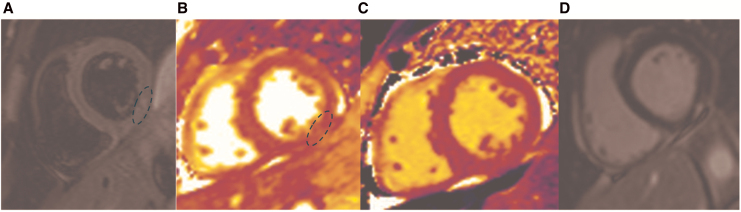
Follow-up cardiac magnetic resonance imaging. Follow-up CMR showed preserved ventricular size and systolic function, substantial reduction of myocardial oedema compared with baseline, and persistent focal non-ischaemic subepicardial late gadolinium enhancement consistent with residual post-inflammatory scar. Region-of-interest analysis was used to further characterize the residual signal alteration on T2-weighted imaging and T2 mapping. *(A*) T2-weighted STIR sequence. Mid-ventricular short-axis view showing markedly reduced myocardial hyperintensity compared with baseline, with mild residual patchy signal alteration in the inferolateral wall (ROI). *(B*) T2 mapping (T2-prepared bSSFP). Mid-ventricular short-axis view showing normal global T2 values (43 ± 5 ms), with a mildly increased focal T2 value at the ROI in the inferolateral wall (50 ± 3 ms), consistent with a possible mild residual regional abnormality. *(C*) Native T1 mapping (MOLLI sequence). Mid-ventricular short-axis view demonstrating normal global native T1 values, indicating resolution of diffuse myocardial injury. *(D*) Late gadolinium enhancement (PSIR gradient-echo sequence). Mid-ventricular short-axis view demonstrating persistent focal non-ischaemic enhancement with subepicardial distribution in the basal inferior and inferolateral segments of the left ventricle, compatible with residual post-inflammatory myocardial scar.
